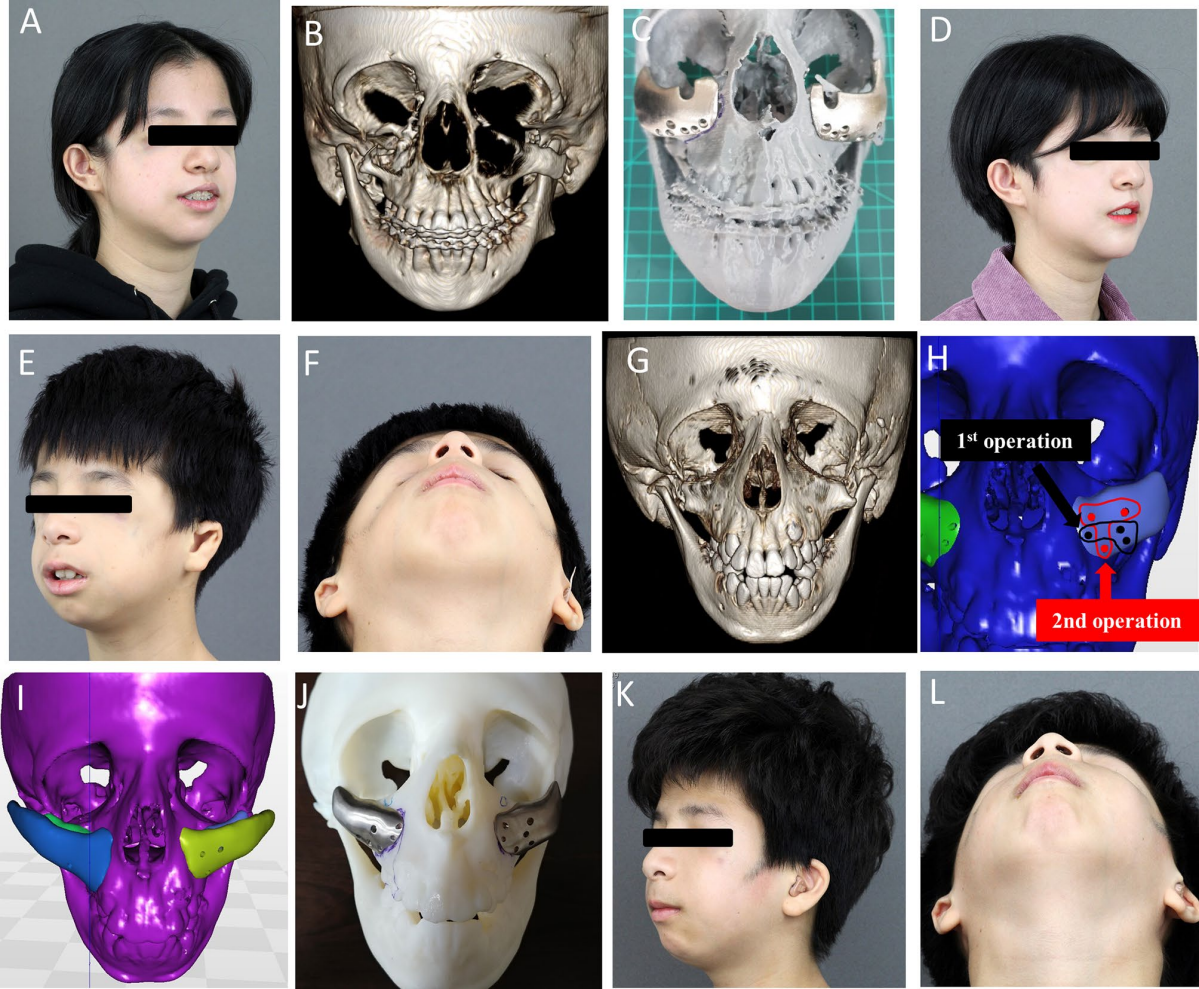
Photographs of patients with Treacher-Collins syndrome. (A) An 18-year-old woman with a depressed midface and downward lateral canthus and eyelids. (B) A three-dimensional (3D) rendering radiography of the facial skeleton. (C) A custom-made 3D-printed zygomatic implant. (D) Facial photographs before and 12 months after surgery. The volume of the orbital and zygomatic area increased. The patient has a normal face after simple surgery; (E, F) Facial appearance of a 9-year-old boy with Treacher-Collins syndrome. (G) Underdeveloped zygoma and orbital bone. (H, I) Considering growth, only three holes out of six were used to fix the zygomatic implant. The other remaining three holes were used for the second surgery. (J) A titanium 3D-printed zygoma was fabricated. (K, L) Facial photographs, taken 3 months after surgery, show that the volume of the zygoma and orbital area increased.
